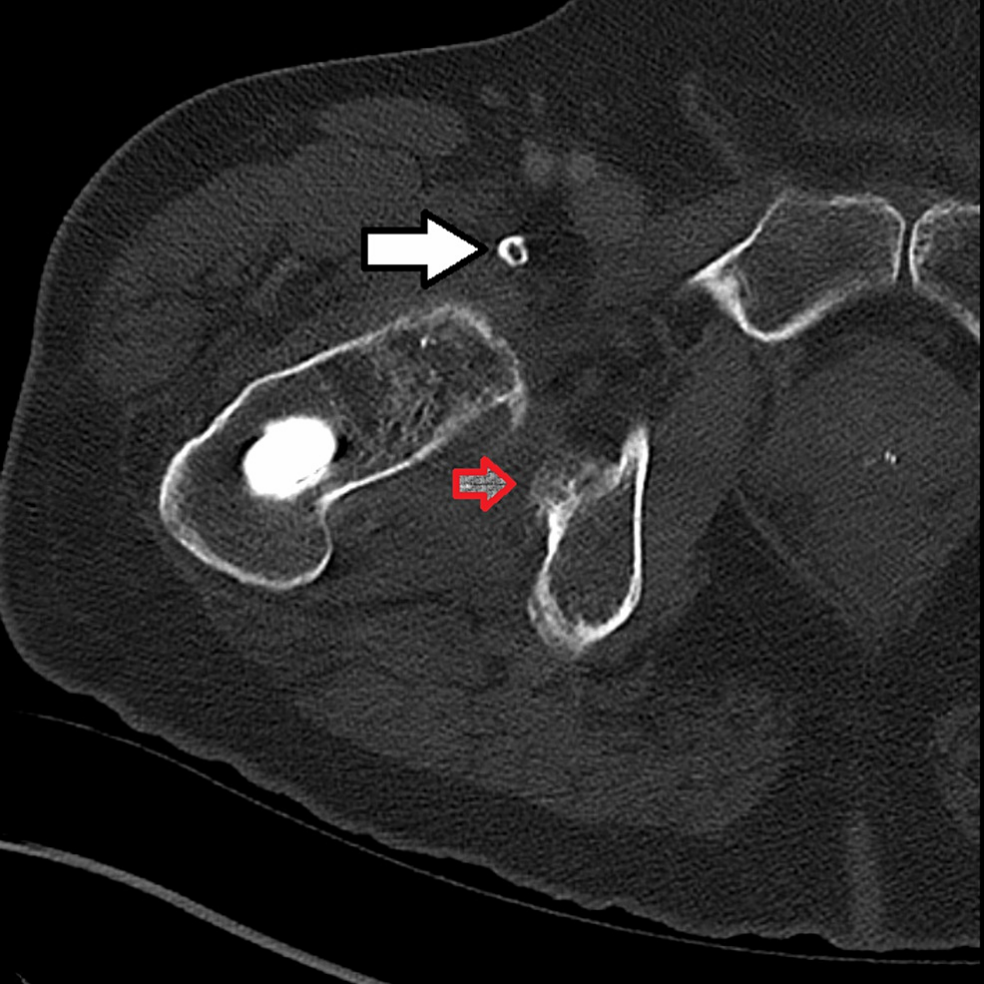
CT image of the right hip, axial view The right hip shows evidence of a 3.2 x 1.4 x 1.2 cm well-corticated ossification involving the lateral acetabulum (red arrow) and calcification of the iliopsoas tendon with a 2.9 x 2.6 x 1.6 cm region of fluid anterior to the acetabulum iliopsoas bursa (white arrow).
